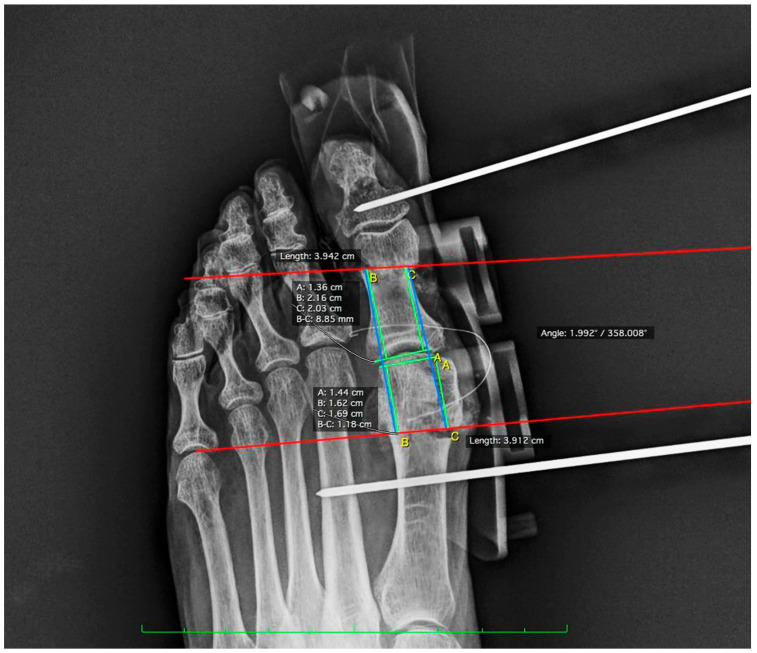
Post-surgical radiographic measurements lines using the Osirix program.
